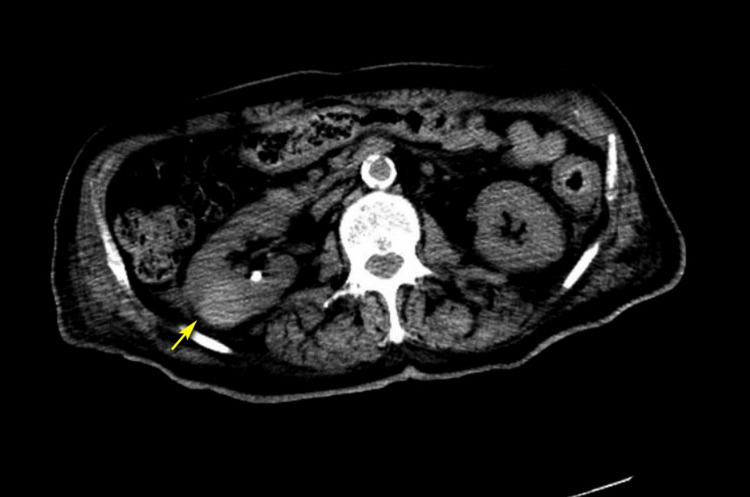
CT scan of the abdomen and pelvis. A hyperattenuating lesion measuring 3.1 × 3 cm was noted in the maximal axial dimension, which was suspicious for a renal mass (yellow arrow). CT: computed tomography
